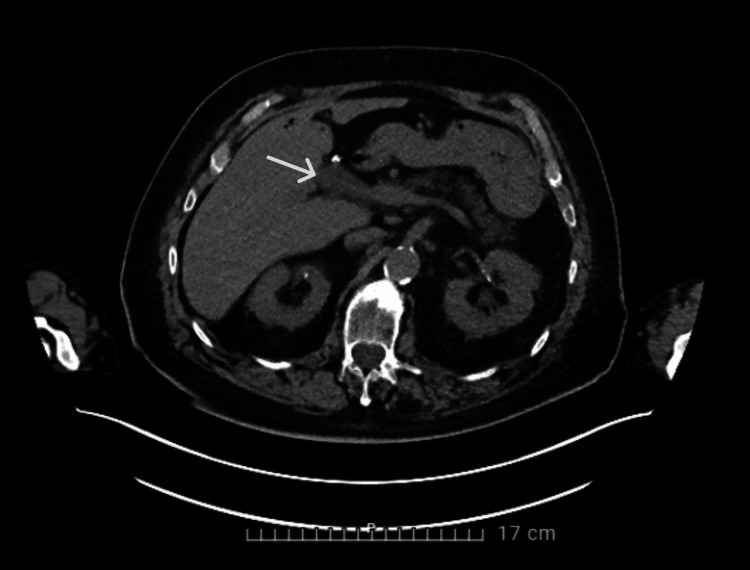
CT of the abdomen showing distension of the portal vein with surrounding soft tissue cuff
